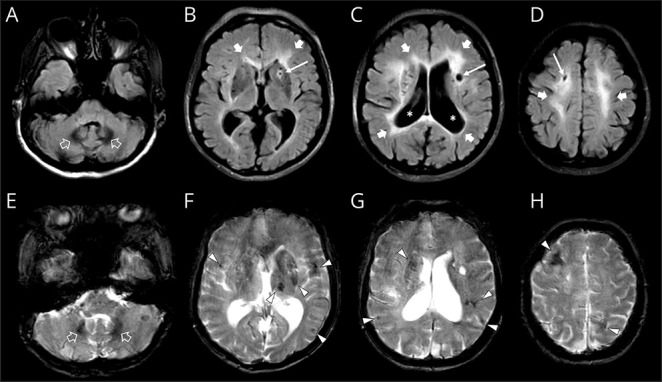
MRI Neuroimaging Findings Brain MRI from Participant 1 at 60 years, with axial Fluid-Attenuated Inversion Recovery (FLAIR) images (A–D) and gradient echo T2*-weighted images (E–F) revealing reduced periventricular white matter volume with consequent enlargement and posterior squared appearance of the lateral ventricles (asterisks). A–D Extensive signal alterations of the frontal and parietal periventricular white matter (thick arrows), also involving the left anterior temporal lobe and external capsules. Small cavitations or lacunar infarcts are noted in the cerebral white matter and basal ganglia (thin arrows). E–F Multiple areas of low T2* signal likely consistent with calcifications at the level of the subcortical cerebral and cerebellar white matter, basal ganglia, and brainstem (arrowheads). The cerebellar dentate nuclei are also likely calcified (empty arrows). Overall, these findings are consistent with a severe vascular leukoencephalopathy.
